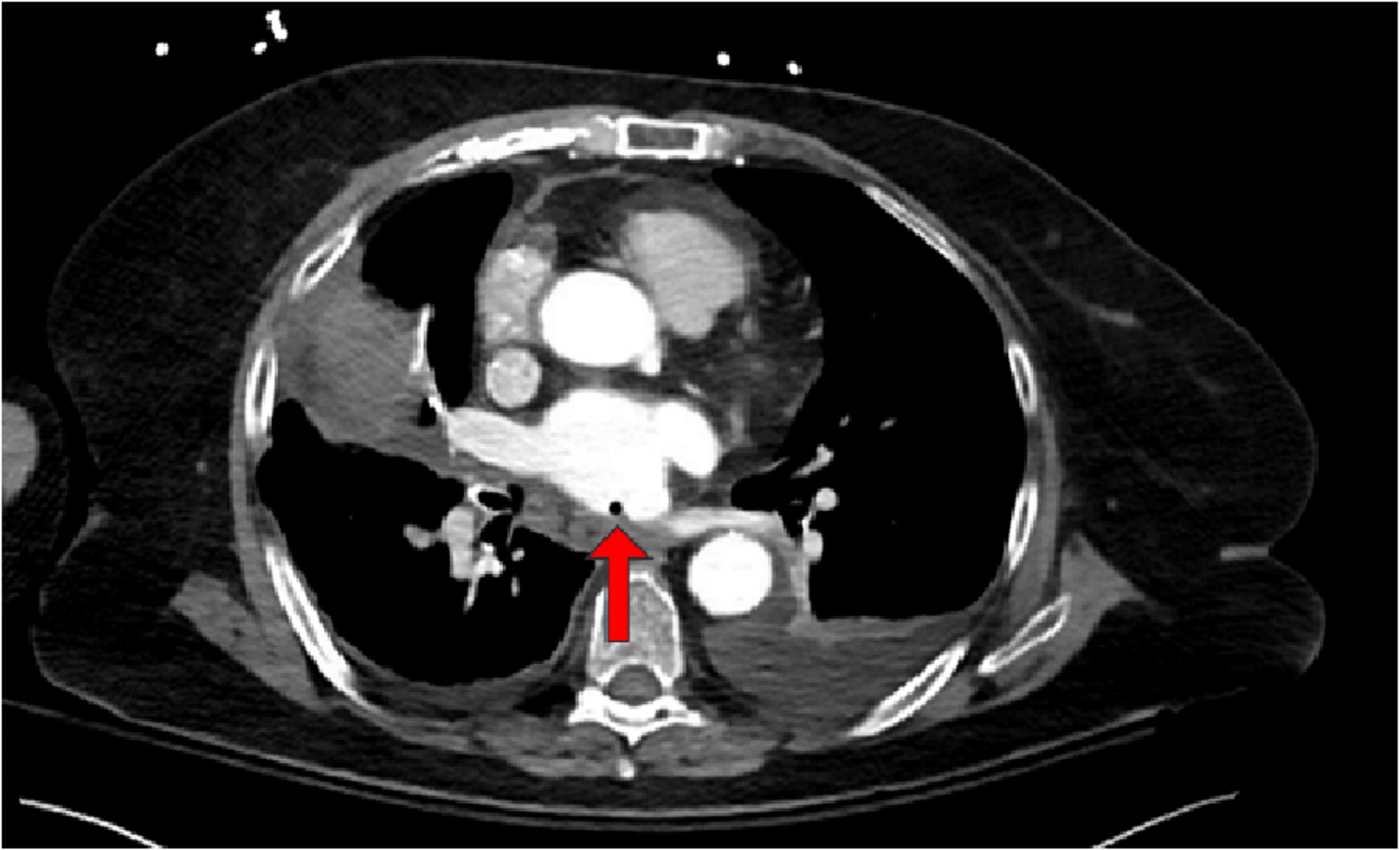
Transverse view computed tomography (CT) of the thorax demonstrating the fistula between the left atrium and the esophagus (indicated by the red arrow)
